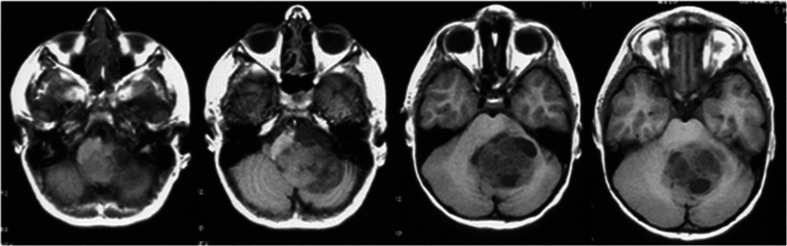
Case 4. Pre-operative T1-weight axial MRI showing a large heterogeneous tumor in the left CMF and CPA displacing the fourth ventricle
